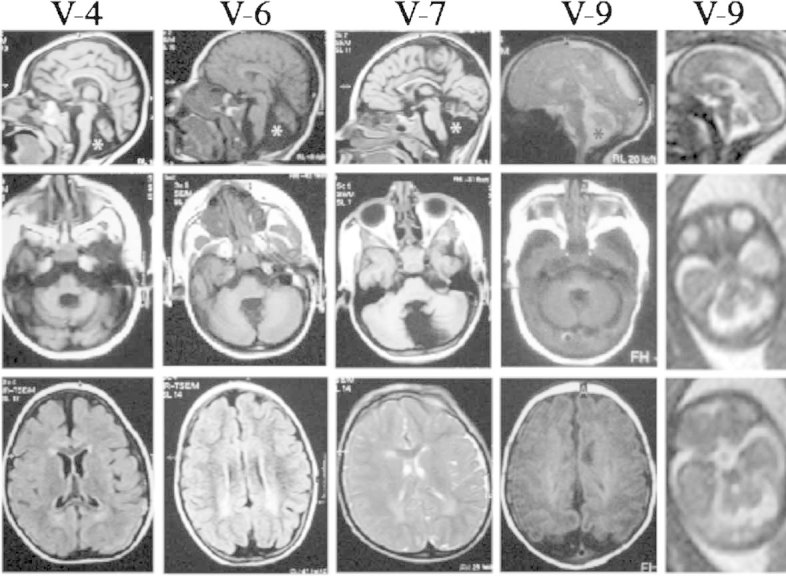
Brain MRI for present patients: First column, patient V-4; second column V-6; third column V-8; fourth column V-9. Top: Brain midline sagittal MR images showing cerebellar hypoplasia/atrophy (arrows) with enlargement of the fourth ventricle (*), thin corpus callosum, and simplified gyral pattern. Middle axial views at the level of the medulla showing failure of the hemispheres to fuse at the midline (arrowheads). Bottom: Axial views at the level of cerebrum, showing simplified gyral pattern and high signal of deep white matter indicating defective myelination. Dysplastic occipital lobe bilaterally is present in both V-6 and 9. Fetal MRI for V-9 (far right) taken at 25 weeks, showing cerebellar hypoplasia on sagittal and axial views.
